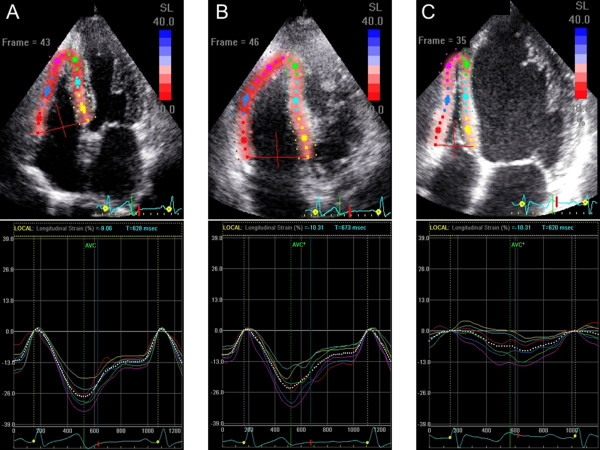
Examples of RV strain imaging in the apical four-chamber view (top) and corresponding time-strain curves (bottom) from the same subjects shown in the Figure4. (A) the normal subject, (B) the patient with OMI in the territory of left anterior descending artery and low plasma BNP level, and (C) the patient with OMI in the all 3 coronary artery territories and high plasma BNP level.
